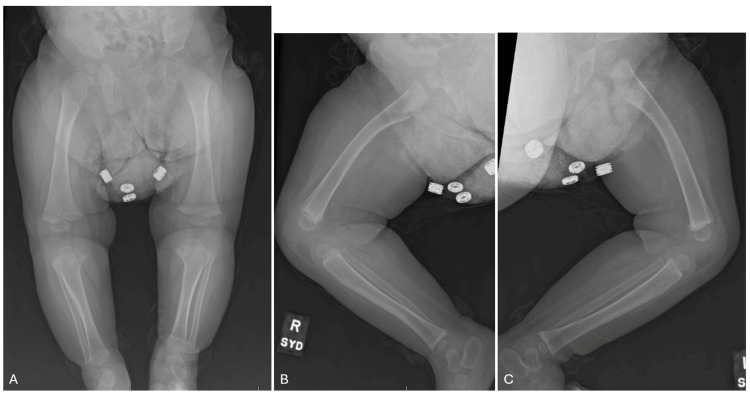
Anteroposterior (A), right lateral (B), and left lateral (C) radiographs at one year of age. The long bones appear normal with no periosteal reaction.
